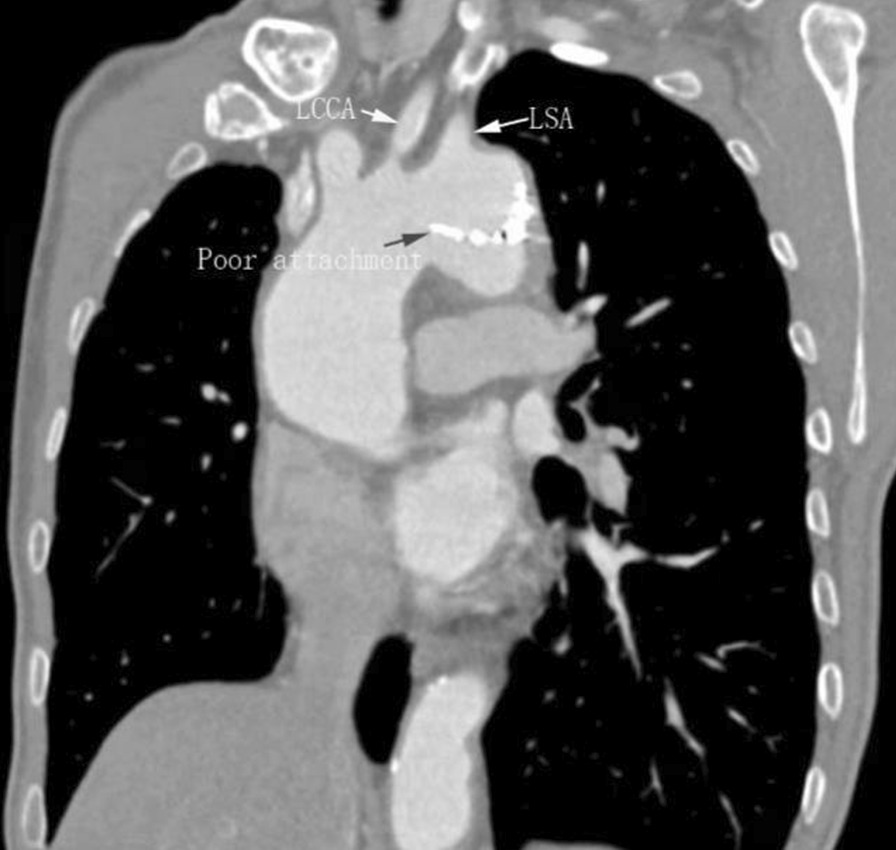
Obliquely reconstructed CTA one year later demonstrated that the proximal stent was poorly attached to the concave wall of aortic arch. LSA: left subclavian artery, LCCA: left common carotid artery
